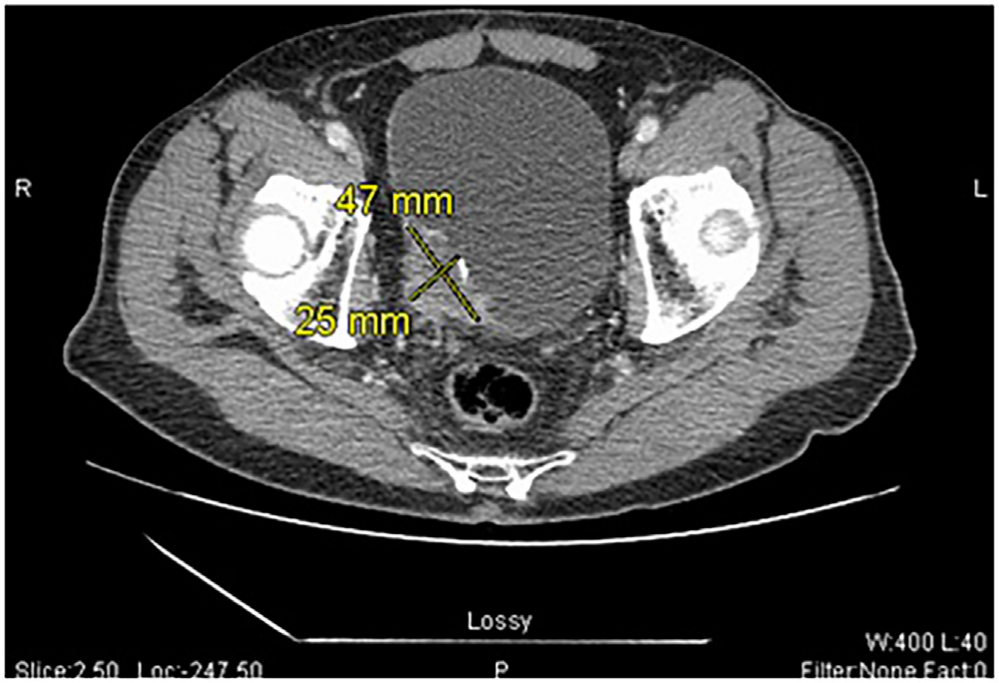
Axial computed tomography (CT) views of the incidental 2.5 × 4.7 cm mass. The mass is located in the right, posterior bladder, and has irregular margins with nonhomogeneous soft-tissue density.
